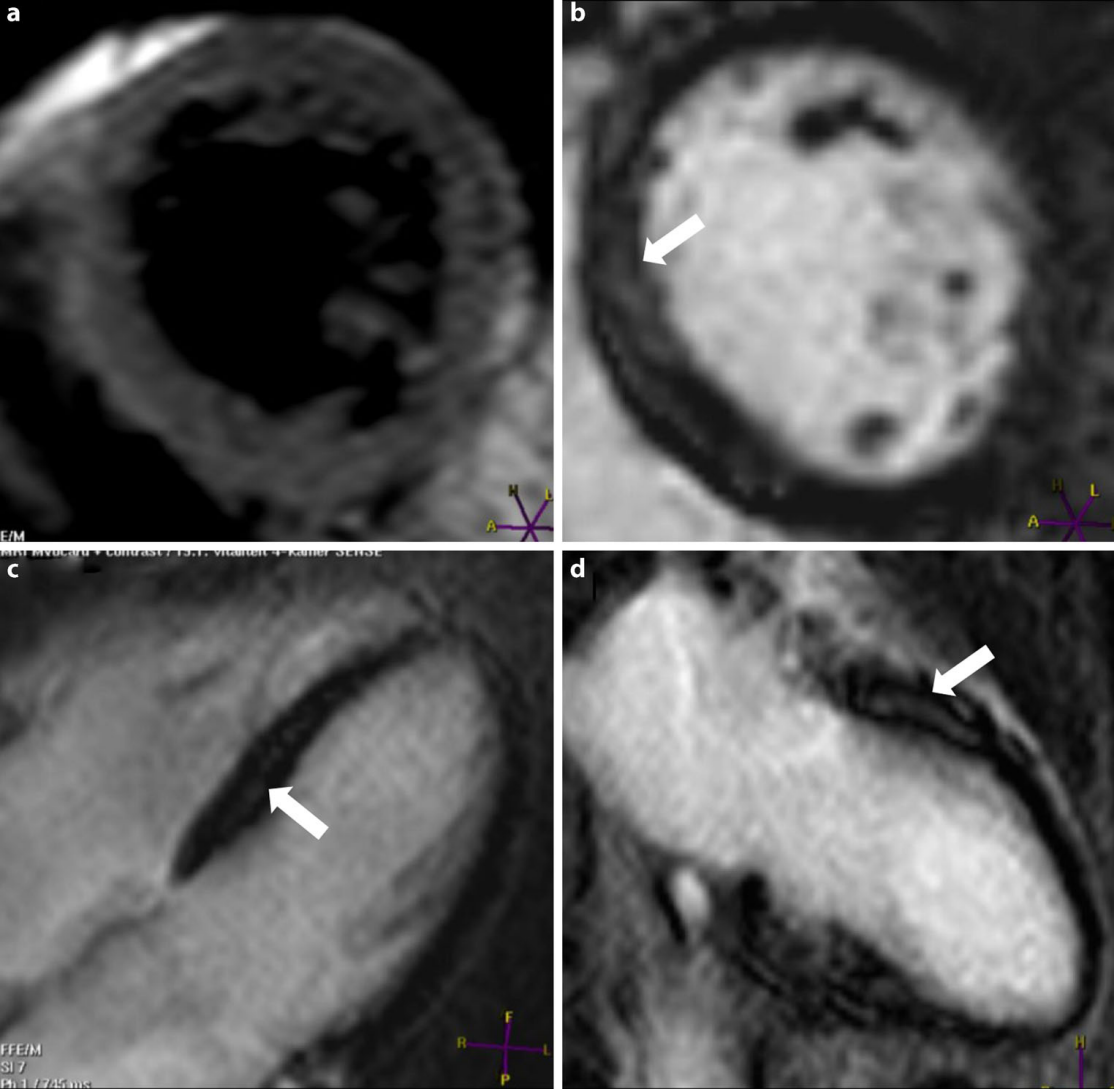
CMR findings in patient with chronic fatigue syndrome. Patient 1. a T2-weighted CMR imaging short-axis view; no presence of increased signal intensity was observed. b, c, d Contrast-enhanced-CMR imaging short-axis view, four-chamber view and two-chamber view; arrow demonstrates midwall contrast enhancement in the basal inferoseptal, septal and anteroseptal segments
